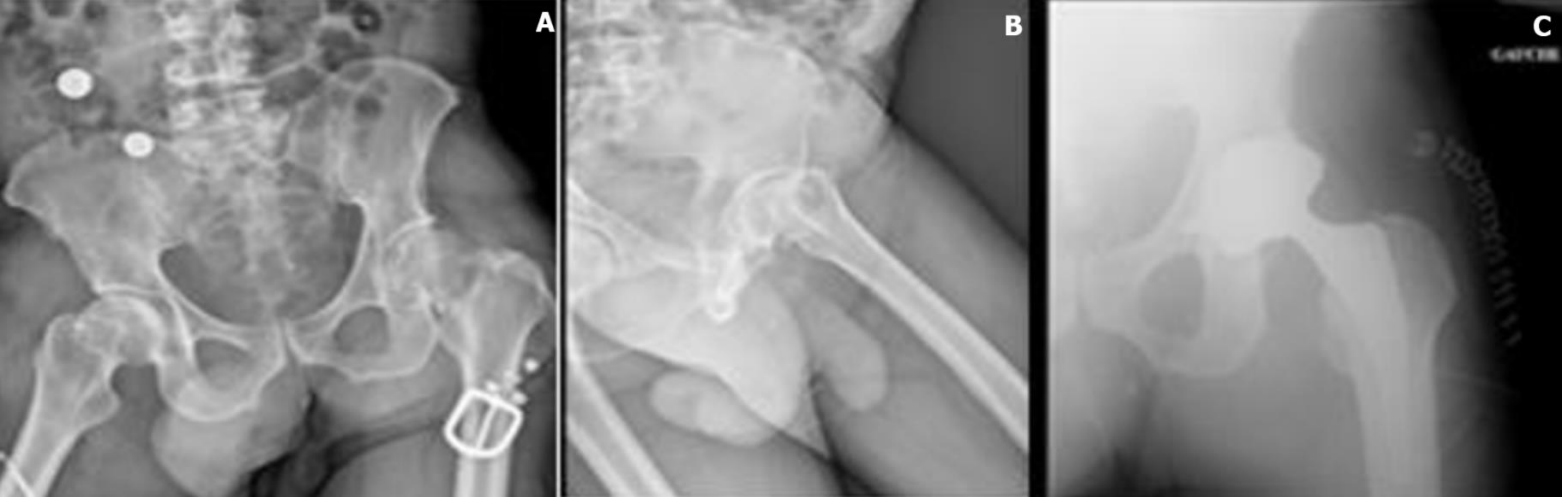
(A,B) radiographies préopératoires et (C) postopératoire
